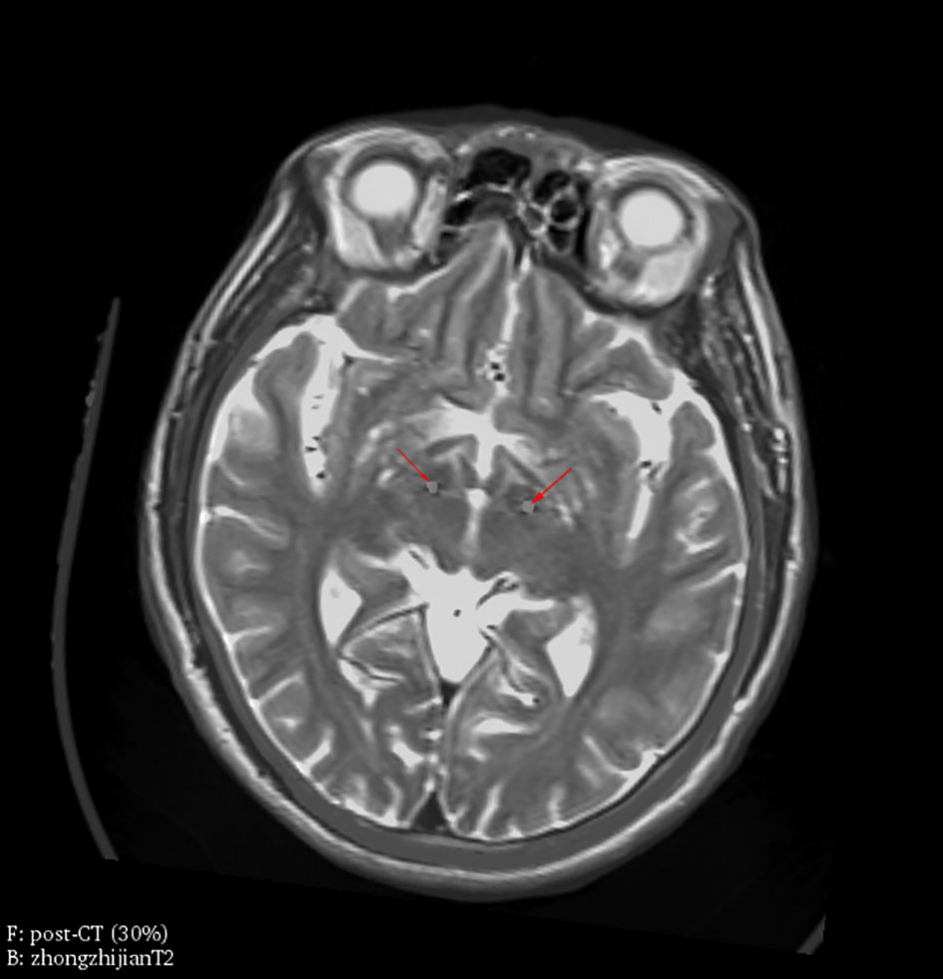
Electrode location was verified by fusion images of postoperative CT and preoperative MRI. Electrodes were located in bilateral STN (red arrowheads) in one representative patient, which is confirmed by fusion images of postoperative CT (30% transparency) as foreground and preoperative MRI (T2, 0% transparency) as background.
